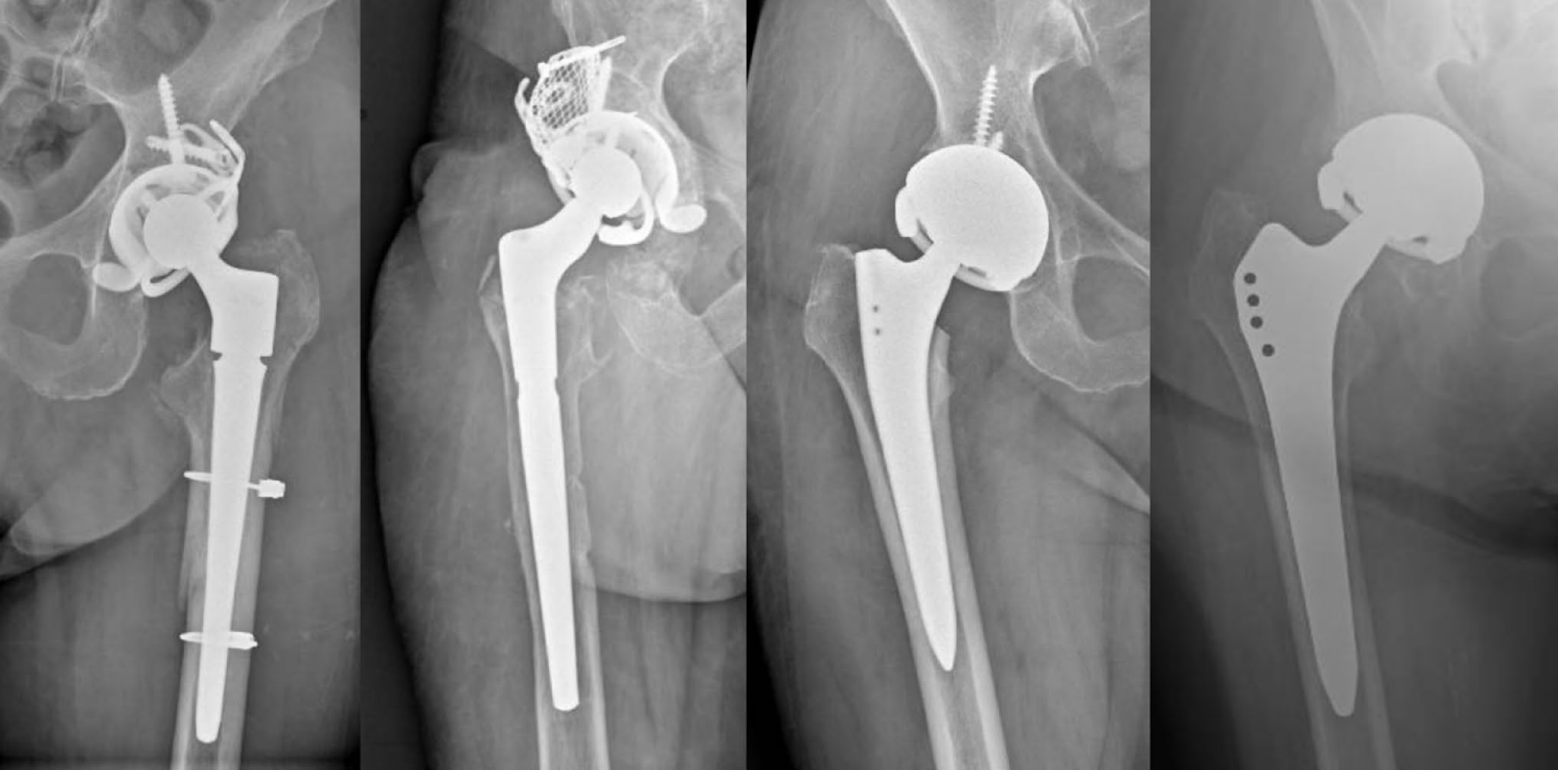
Series of X-rays at follow-up: some types of implants with constrained acetabular liners
